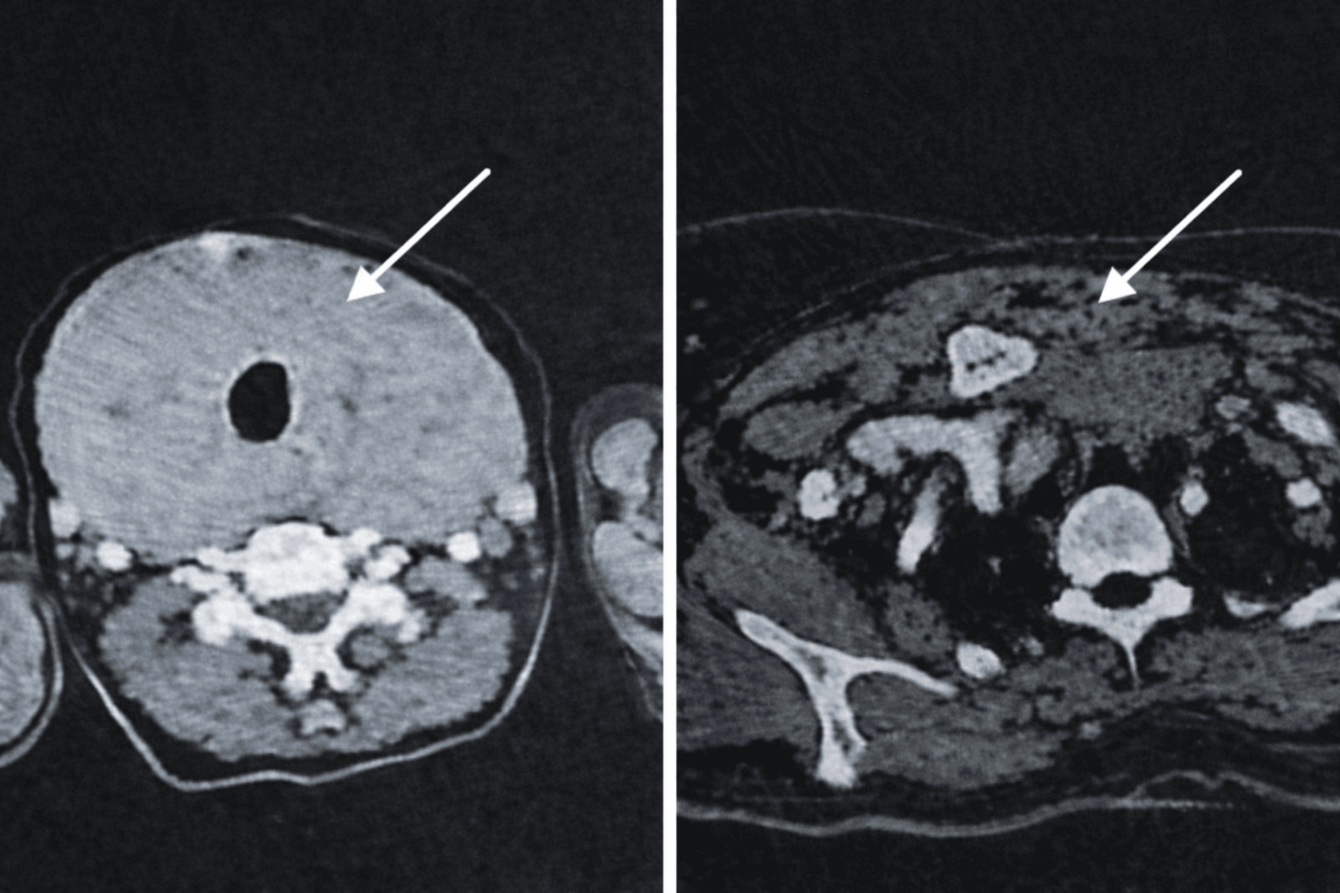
Axial cervicothoracic computed tomography scan demonstrating a voluminous plunging goiter arising from the thyroid gland and extending into the mediastinum White arrows indicate the location of the goiter in the cervical and mediastinal regions, showing its extension and mass effect on surrounding structures.
